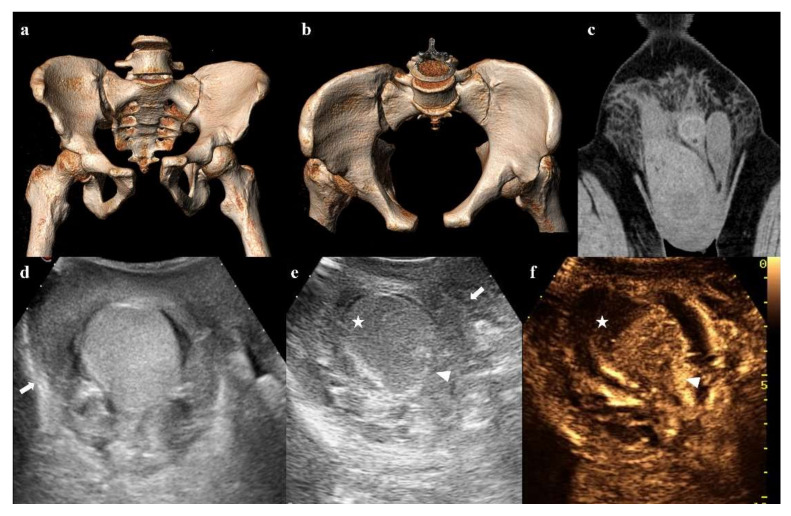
Three-dimensional volume-rendering CT reconstructions in AP and inlet views show an anterior–posterior compression fracture (a,b). Coronal CT image shows hematoma in the right inguinoscrotal region (c). Ultrasound shows an inhomogeneous hematoma on the extra-albuginea side (arrow in (d,e)) and a hypoechoic area in the upper part of the testis (star in (e)). Contrast-enhanced ultrasonography (CEUS) image clearly shows that the hypoechoic area is avascularized, as a hematoma (star in (f)). Ultrasound and CEUS also show the irregularity of the testicular contour (arrowhead in (e,f)). These findings are suggestive of testicular rupture.
